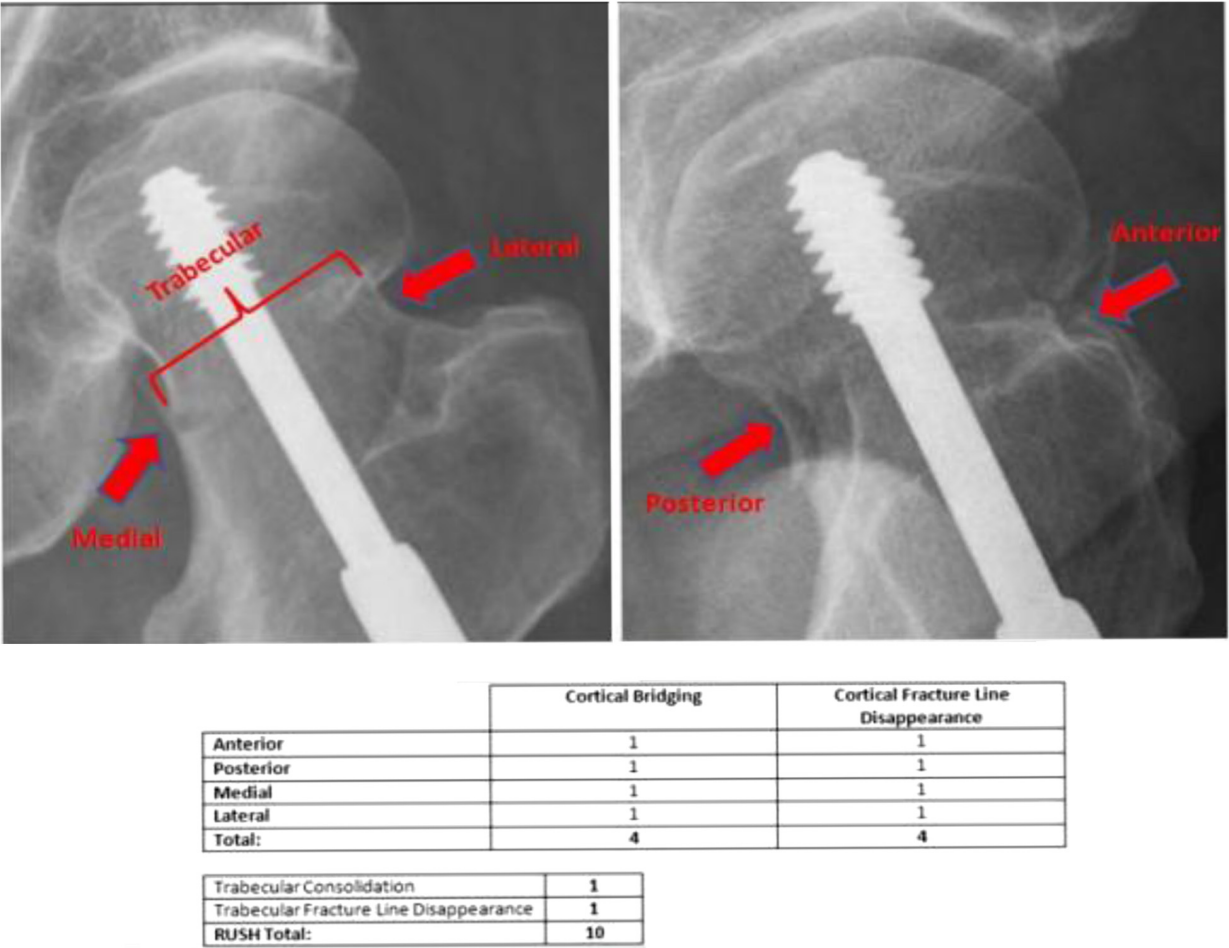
Early post-operative radiographs and RUSH assessment.
